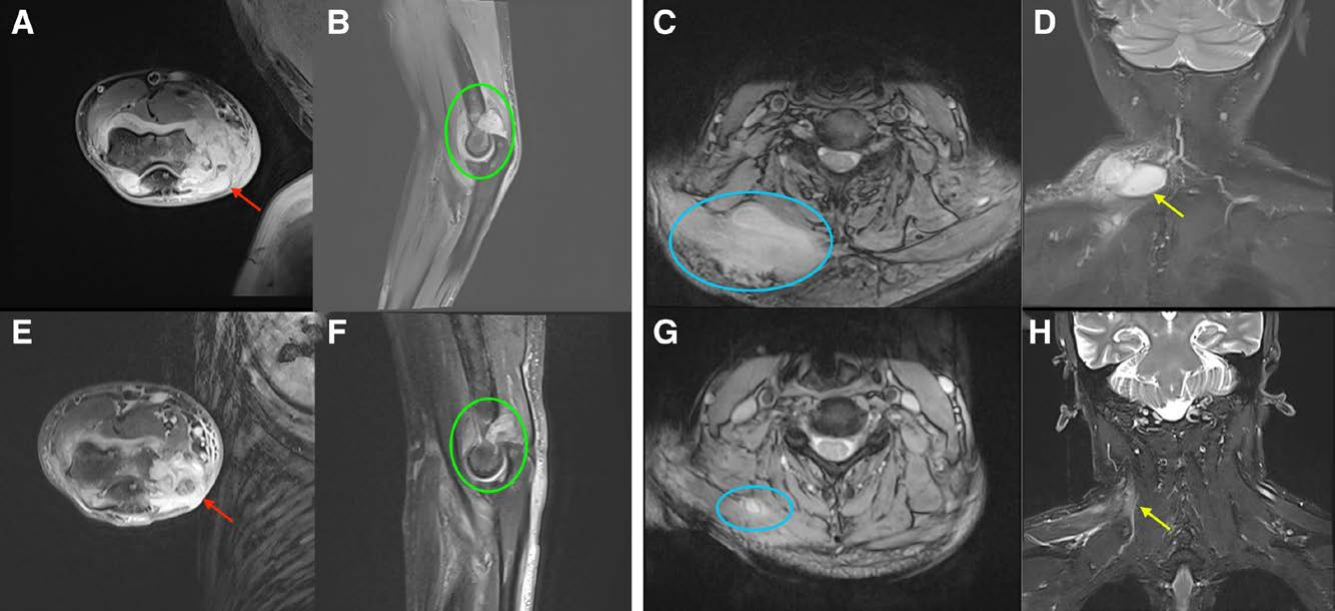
Patient MRI results. (A and B) Inflammatory edema of the right elbow’s distal, radial, and ulnar humeri, possibly due to osteomyelitis; inflammation and exudation of the soft tissues around the elbow joint, with abscess formation; multiple fluid and pus accumulations in the elbow joint cavity and bursa; (C and D) infectious lesions were suspected in the subcutaneous area of the right back of the neck with patchy T2WI high signals; (E and F) abscesses on the right elbow were reduced following therapy; (G and H) neck post-treatment results showed considerable improvement in infectious lesions. (Red arrows and green circles show right elbow alterations before and after infection treatment; yellow arrows and blue circles show neck changes) MRI = magnetic resonance imaging.
